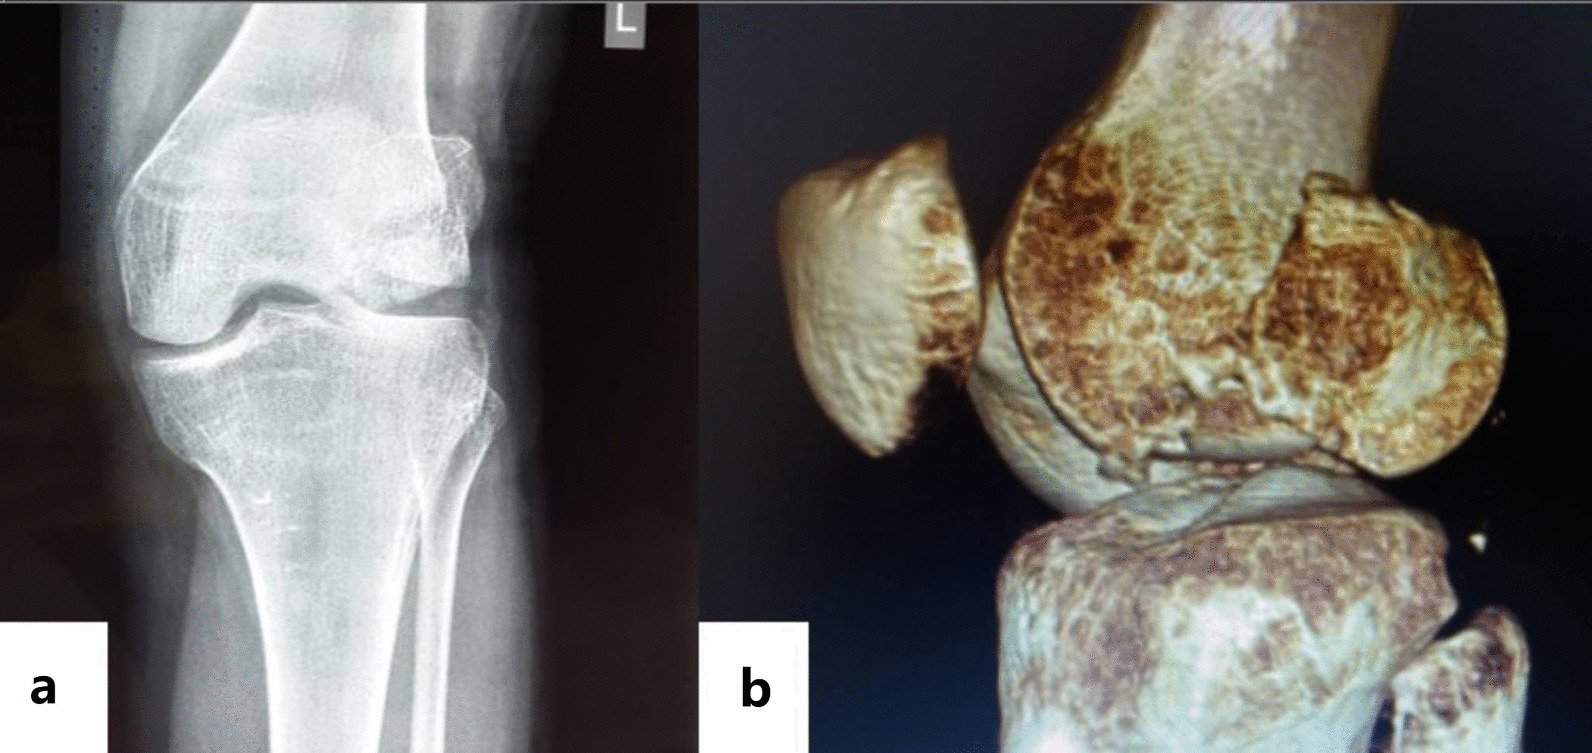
Preoperative X-ray (a) and CT showed severe fracture collapse (b)
